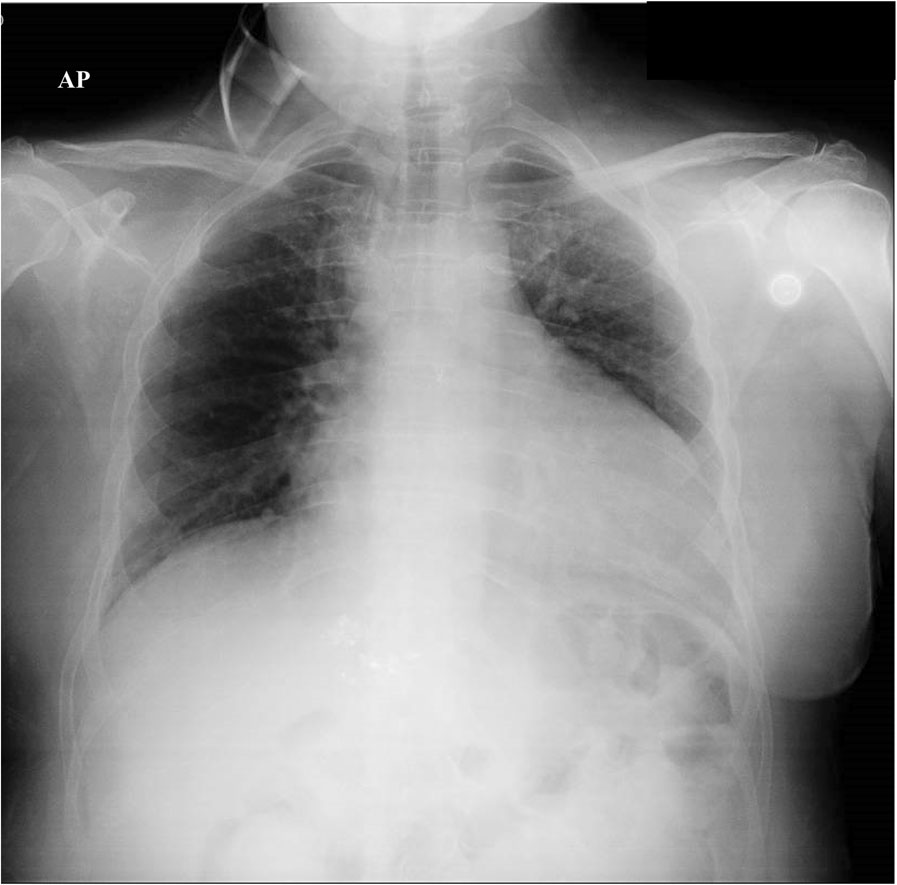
Widespread bronchial wall thickening and enlargement of the cardiac silhouette on chest X-ray from large pericardial effusion
